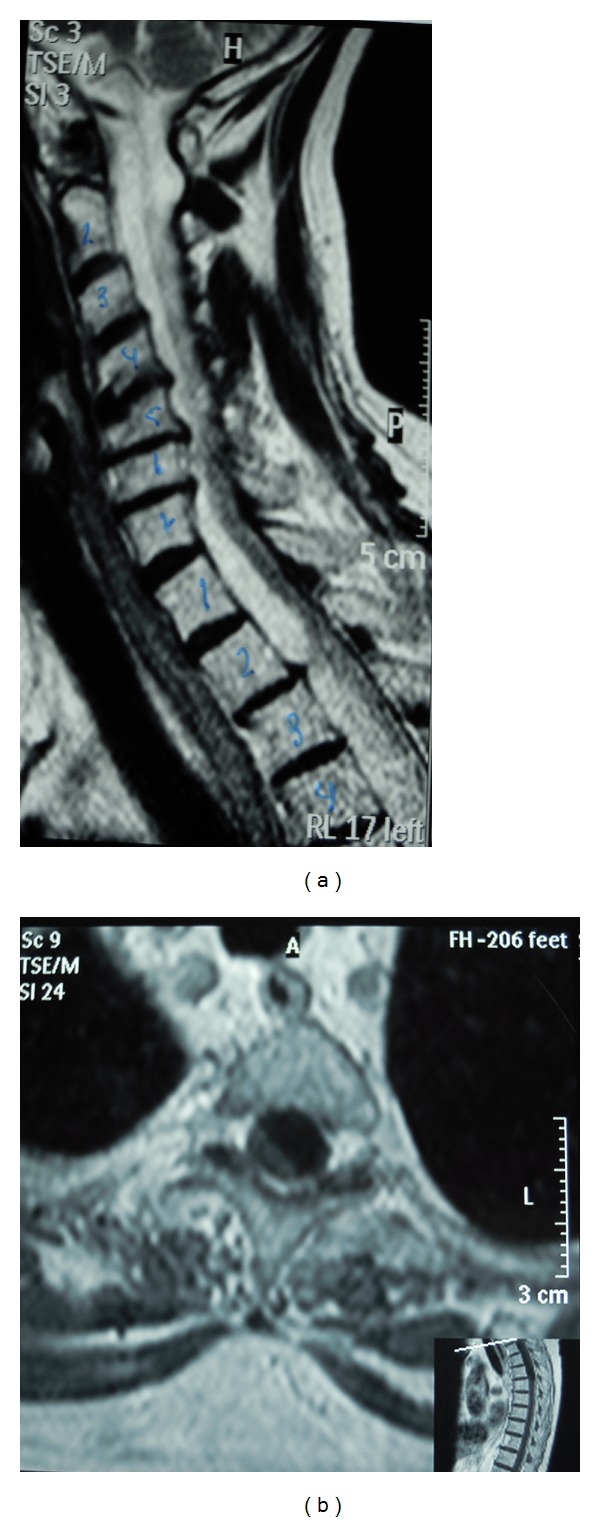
T-2 weight sagittal (a) and T-1 weight axial (b) MRI showing the cervicothoracic SCSH. A severe cord compression is observed.
